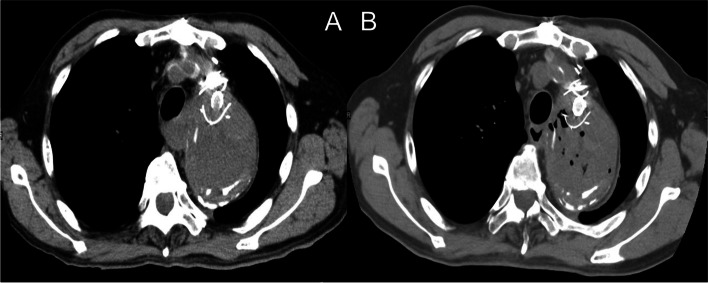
A Computed tomography demonstrates the possibility of stent graft infection complicated by abscess formation around the aortic aneurysmal sac. The aortic arch aneurysmal sac expanded as compared with that 8 months ago. There was no evidence of endoleak. B Plain CT 5 months after the initial admission shows newly developed air inside the aneurysmal sac
